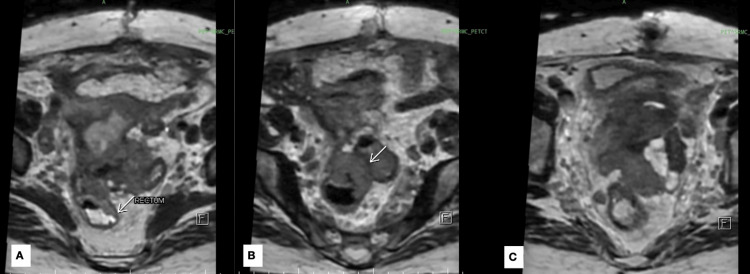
Postoperative magnetic resonance imaging of the pelvis A) Axial T2-weighted (T2W) image showing an ill-defined heterogeneous mass lesion arising from the upper rectum and sigmoid colon from 11 o'clock to 3 o'clock position. The mass lesion is noted extending anteriorly, infiltrating the posterior wall of the uterus; B, C) Axial T2W image showing a few of the adjacent small bowel loops adherent to the above-mentioned mass lesion.
